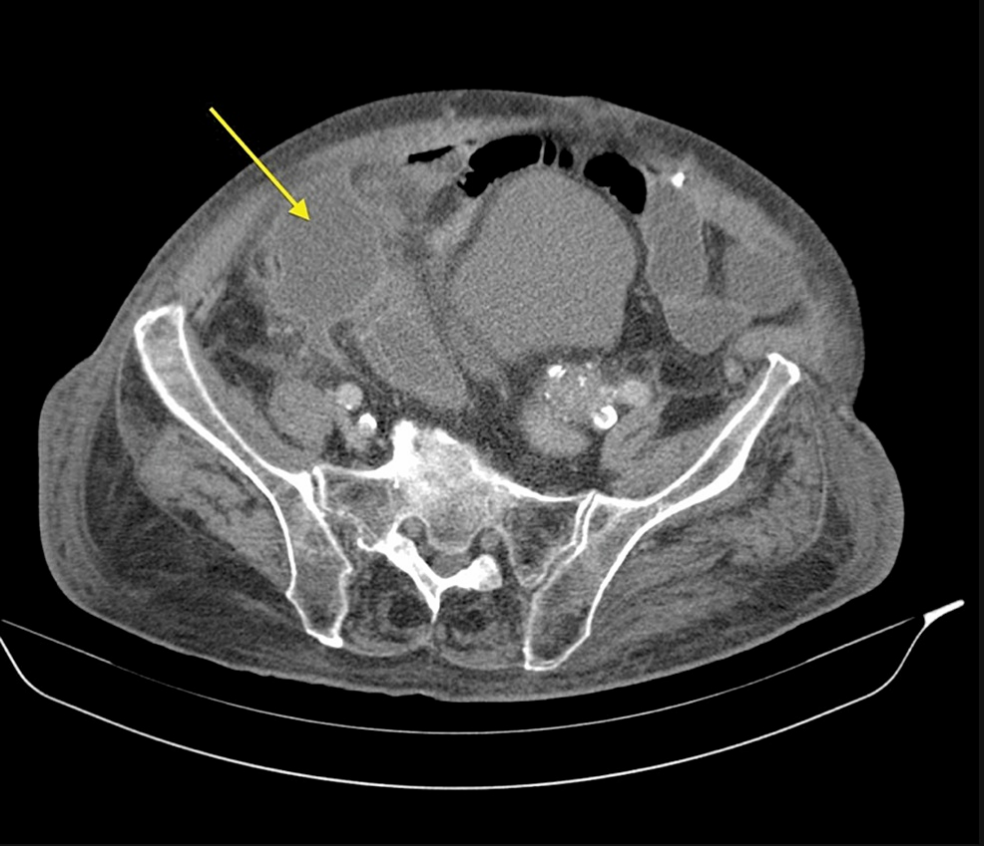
CT scan showing perforated gallbladder (arrow) CT: computed tomography
